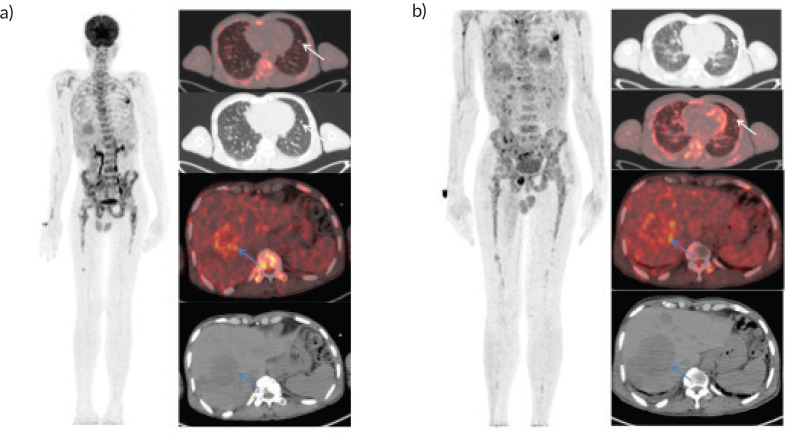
Whole body FDG PET scan. (a): Pretherapy PET/CT shows increased tracer uptake in the thoracic, liver and multiple skeletal regions in maximum intensity projection image (A).Trans-axial fused PET/CT and CT sections shows multiple random distribution parenchymal nodules in the lungs (white arrows) and large necrotic peripheral FDG avid lesion in segment VII/VIII of the liver measuring 5.3 × 5.5 cm .Also noted FDG avid lytic lesions noted in D10 vertebra (blue arrows). (b): Post-therapy PET/CT shows increased tracer uptake in the thoracic, liver and multiple skeletal regions in maximum intensity projection image (A).Trans-axial fused PET/CT and CT sections shows multiple random distribution parenchymal nodules in the lungs(white arrows) and large necrotic peripheral FDG avid lesion in segment VII/VIII of the liver measuring 7.3 × 6.1 cm. Compared to pretherapy PET/CT scan there is increase in size of liver lesions along with increase in number of lung and skeletal lesions suggestive of progressive disease.
